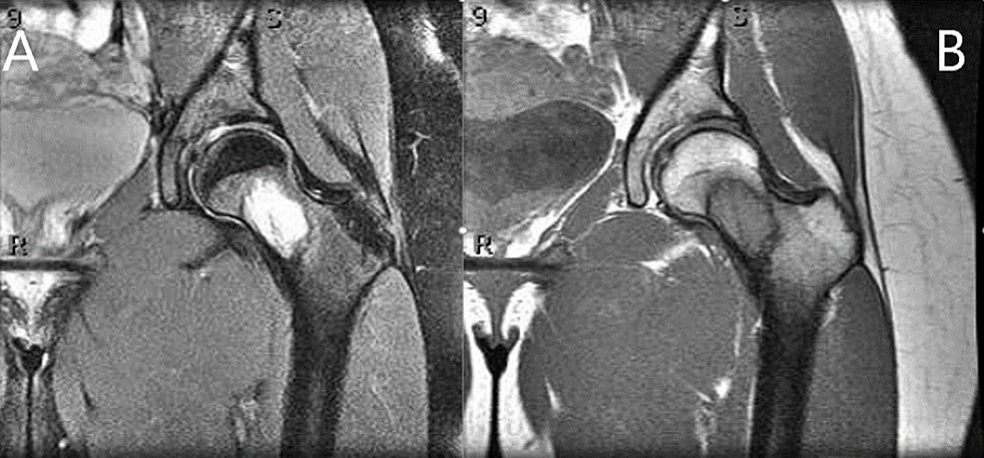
MRI scan of the left hip MRI scan of the left hip with T2-weighted coronal image (A) demonstrating a hyperintense isolated lesion with distinct sclerotic margins and surrounding bone marrow edema within the femoral neck and a T1-weighted coronal image (B) showing an isointense lesion with sclerotic margins
